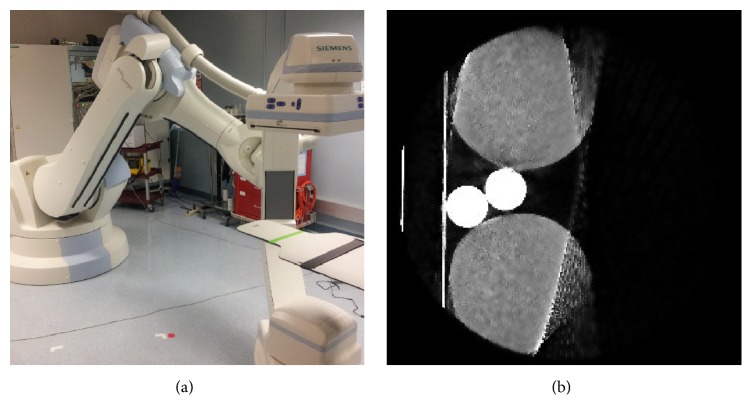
(a) The Siemens Zeego C-arm CT system. The robotic arm allows free movement of the C-arm for scanning patients in standing position. (b) Typical artifacts that arise when scanning knee-shaped objects with a C-arm CT. Due to saturation, the original cylindrical shape is lost, and the front is severely affected by artifacts (window level [−1000, 1500]).
